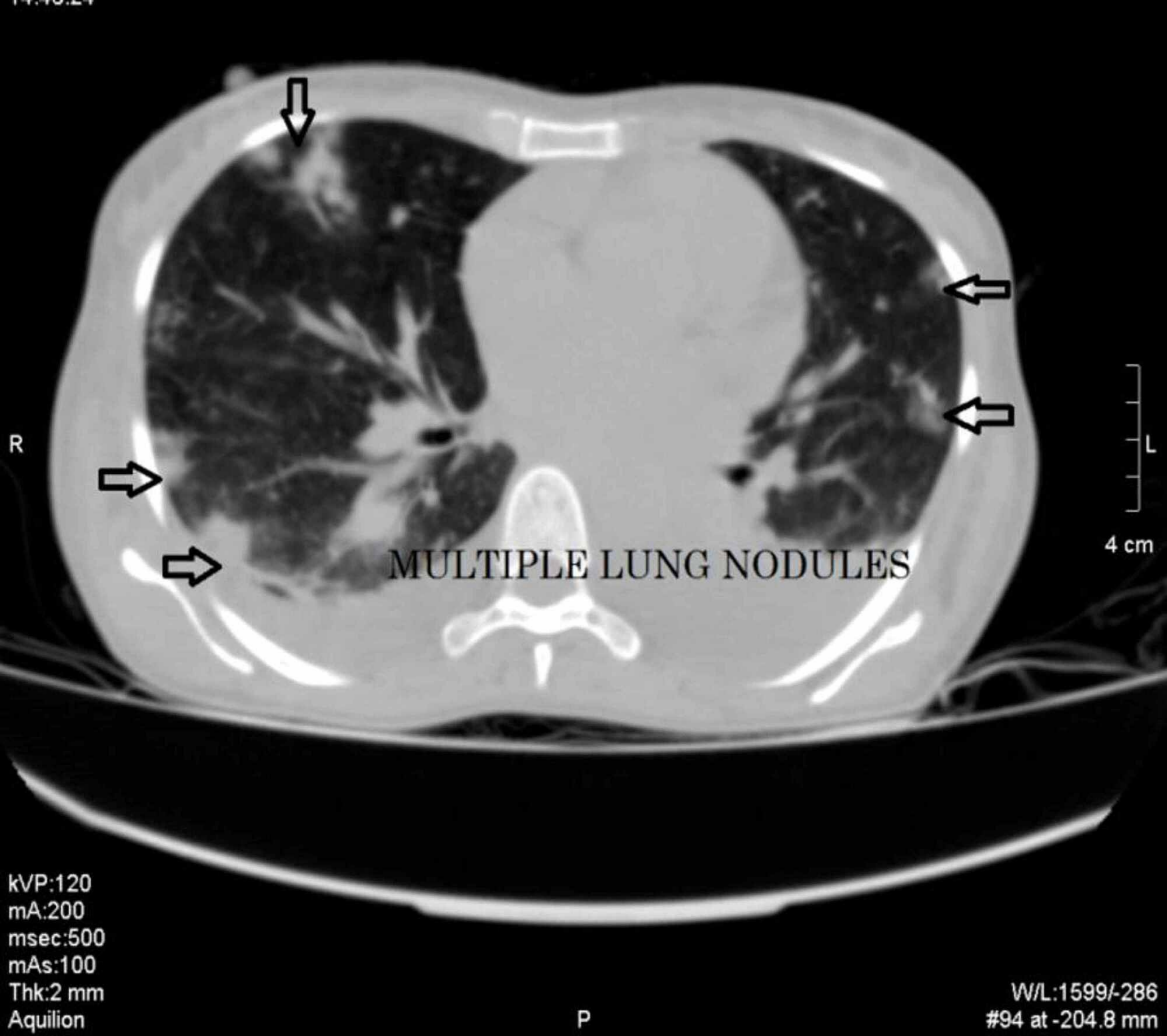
CT chest lung window showing multiple pulmonary nodules and bilateral pleural effusion CT: computed tomography
